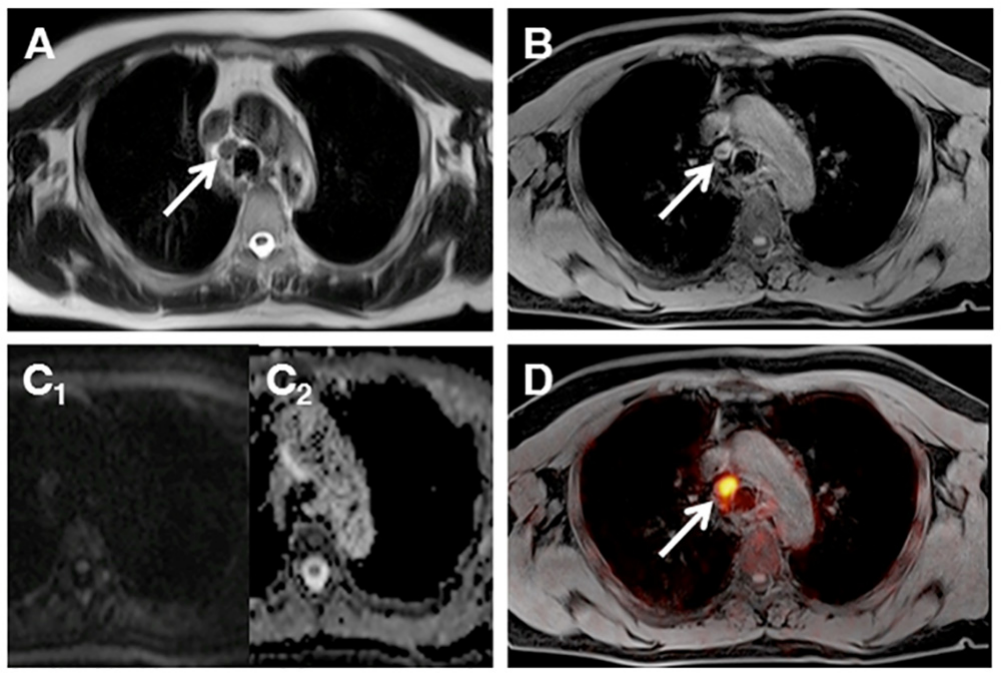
MR images (T2w Haste, a; T1w VIBE, b) show a non-enlarged mediastinal node (arrows), without diffusion restriction (DWI, c1; ADC-map, c2). The identical node shows pathological glucose metabolism and was correctly identified as manifestation of lymphoma with the additional information provided by PET (d).
